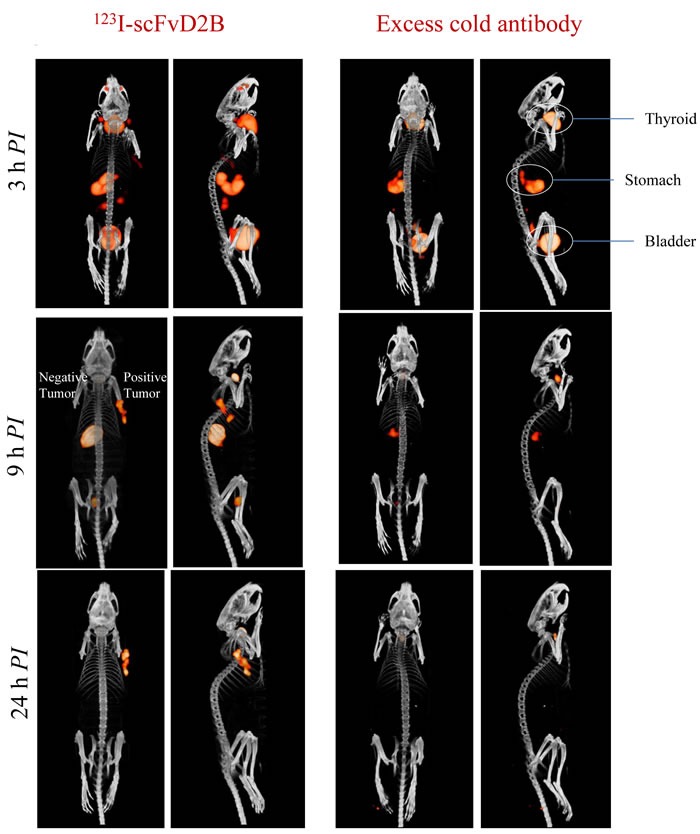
Representative SPECT/CT images in LS174T-PSMA/LS174T model after intravenous administration of 123I-scFvD2B. PSMA-positive tumor LS174T-PSMA (right flank) and PSMA-negative tumor LS174T (left flank) evaluated at 3, 9 and 24 hours post injection. Left: 12 MBq (8 µg; SA = 1500 MBq/mg) administered. Right: 12 MBq administered plus a 100-fold excess of cold scFvD2B.
